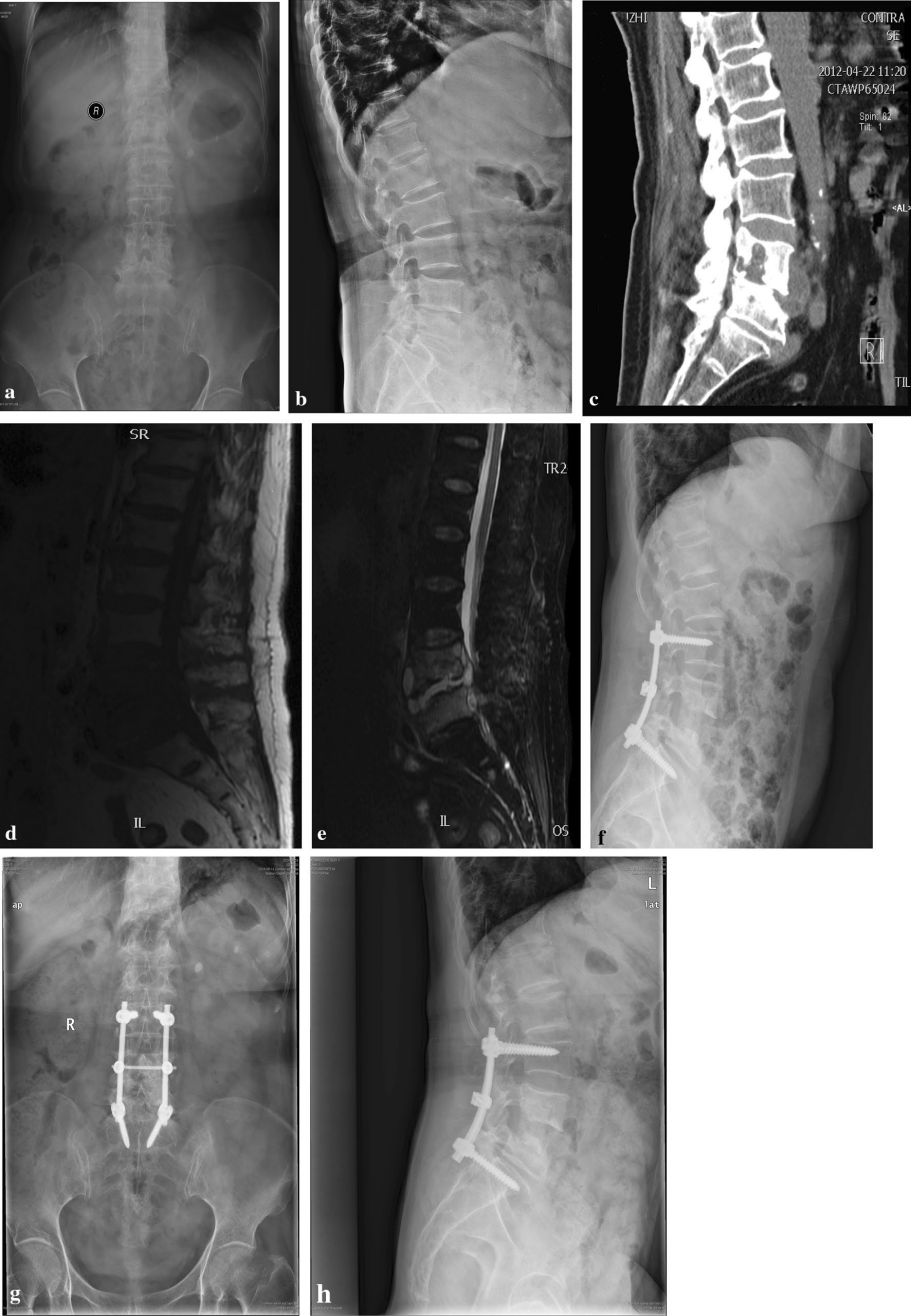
Images in a 64-year-old woman with L4 and L5 tuberculous spondylitis. a, b Preoperative X-ray films demonstrate collapse of the L4 and L5 vertebras with narrow intervertebral space. c–e Preoperative sagittal CT and T1 and T2 W MRIs demonstrate bone destruction and posterior epidural abscess at L4/5. f Immediate postoperative X-film showed that the patient received posterior transforaminal lumbar debridement, interbody fusion and instrumentation at the lesion. g, h X-ray films reveal bone fusion and internal fixation in good position at 2-year follow-up
